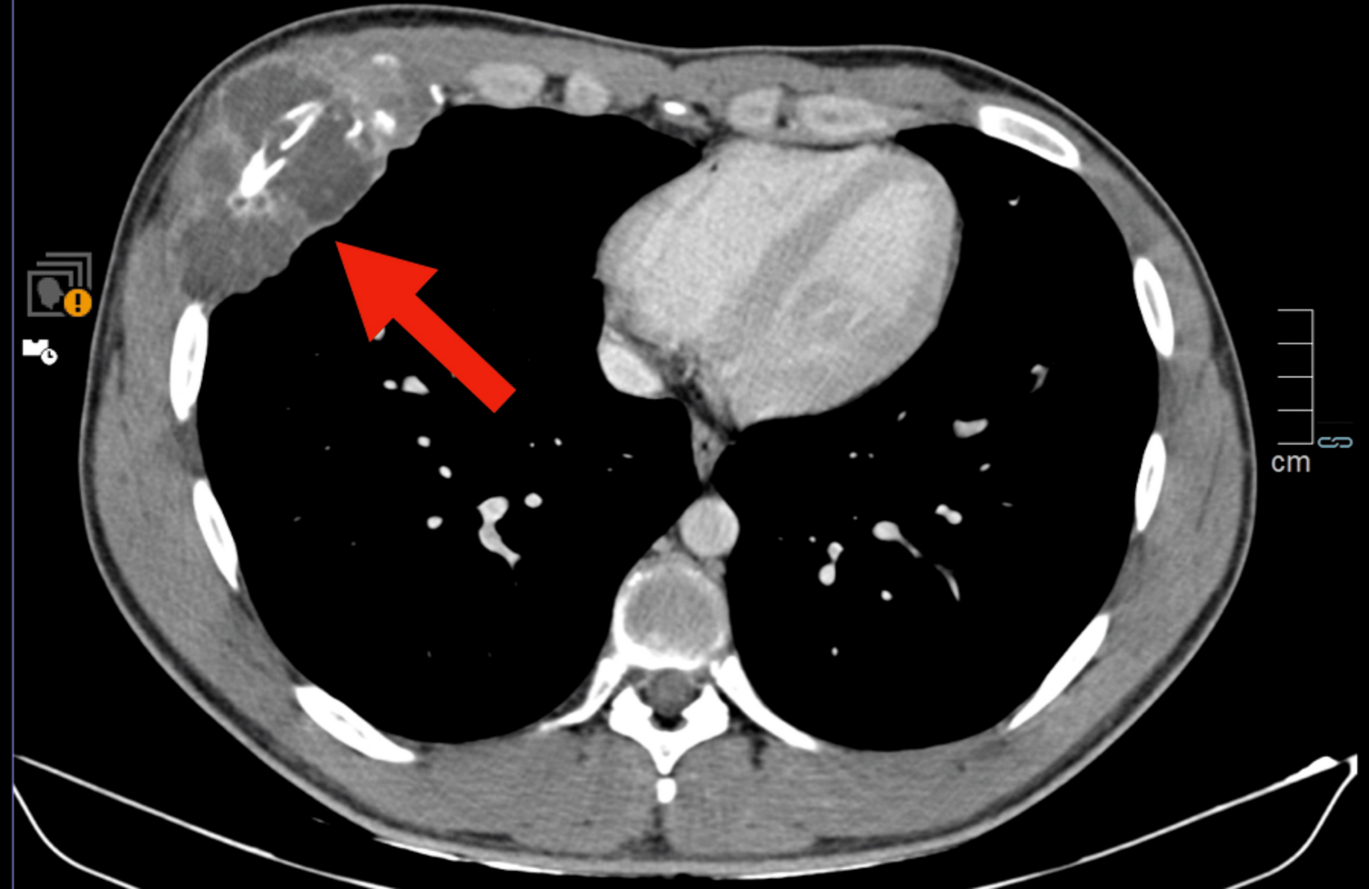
Axial contrast-enhanced CT scan of the thorax Voluminous soft-tissue lytic neoformation infiltrating the anterior aspect of the right sixth rib. This lesion shows heterogeneous contrast enhancement and some peripheral calcifications (red arrow).
